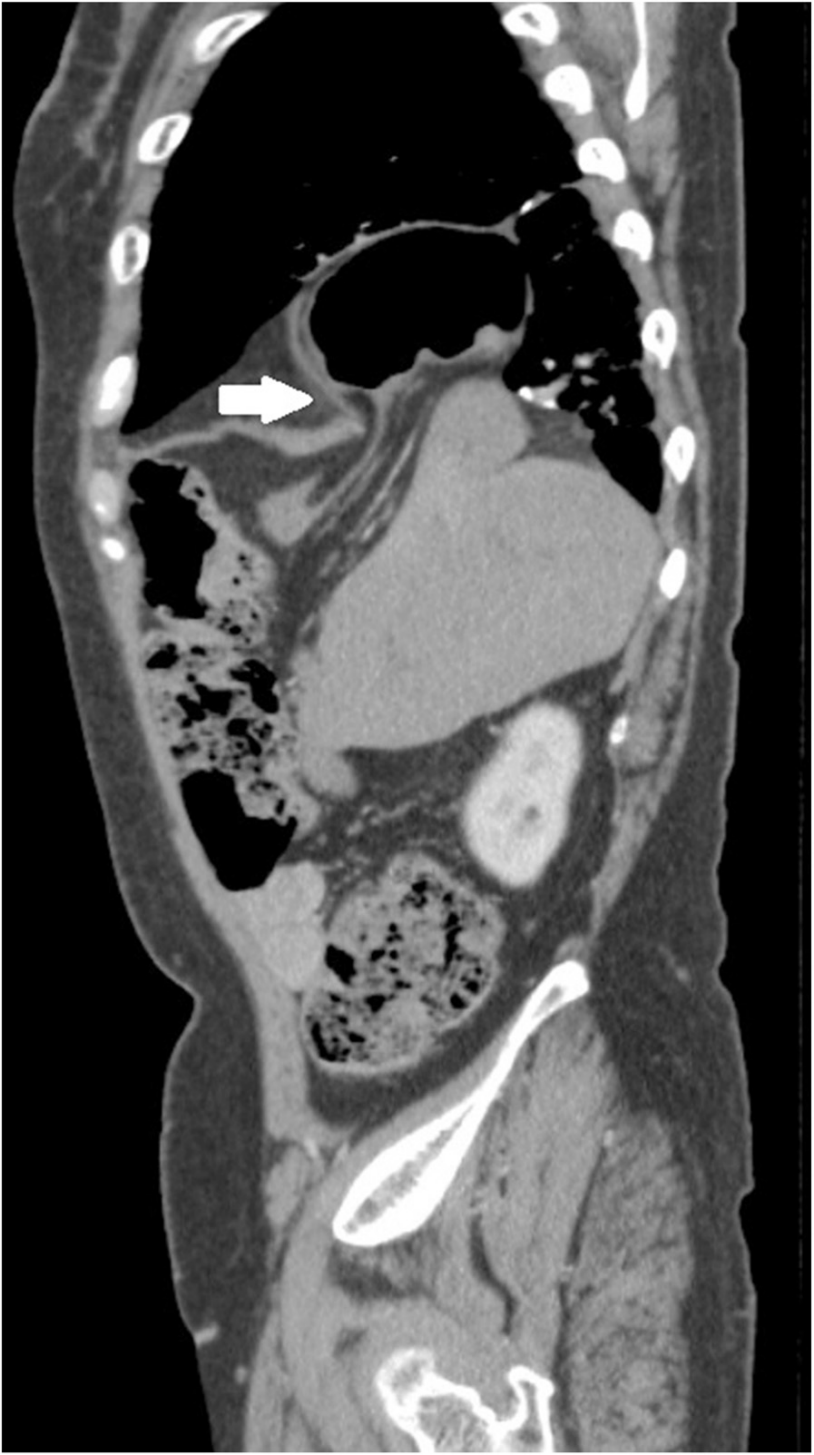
Sagittal sequence of CT shows intestinal incarceration in the right thoracic cavity through the hole through which a part of the liver has herniated (*arrow*)
